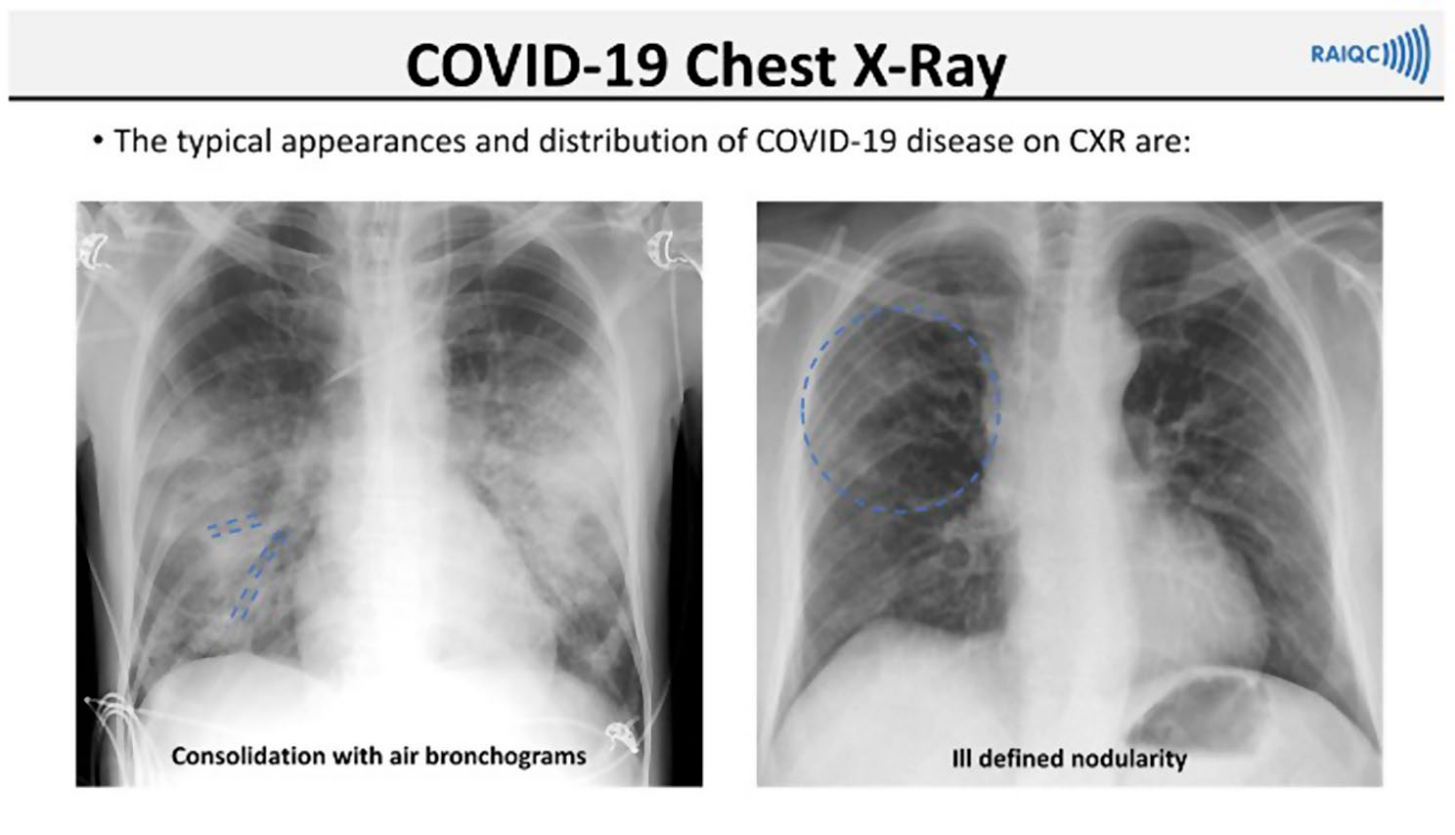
Teaching slide showing typical appearance of SARS-CoV-2 infection on chest X-ray
